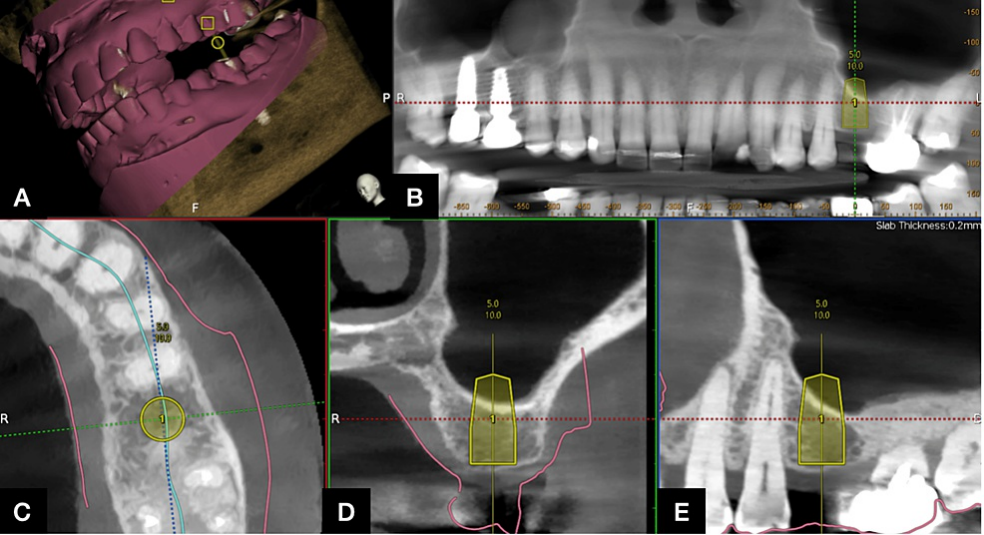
Implant planning under the dynamic navigation software (Navident) (A) Represents the cone beam computed tomography (CBCT) data and the STL files. (B) Implant planning 26 OPG view. (C) Occlusal view of implant position. (D) Bucco-lingual placement of the virtual implant. (E) Mesio-distal placement of the virtual implant
